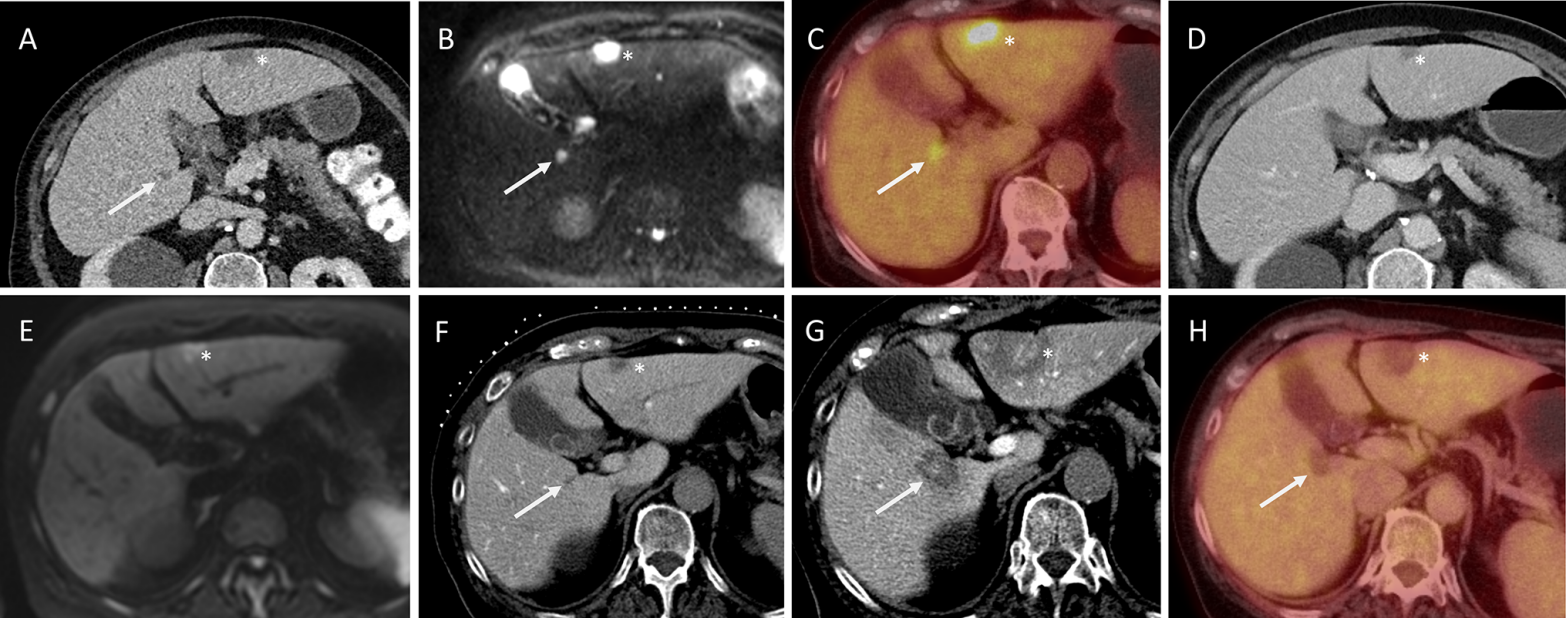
Contrast-enhanced (ce) CT and ceMRI images (A, B) and an 18F-FDG PET-CT image (C) from a 60-year-old men with two colorectal liver metastases in segment III (*) and segment V (white arrow) from a primary rectal carcinoma. After treatment with chemoradiotherapy for the primary rectal carcinoma, the metastasis in segment V disappeared on both ceCT (D) and ceMRI (E). On the intraprocedural CT hepatic arteriography (CTHA), both the metastases in segment III as well as the ‘disappeared’ metastasis in segment V were detected (F) and treated with percutaneous MWA (G) in the setting of the randomized controlled COLLISION trial. Six months after the ablation, there were no signs for local or distant tumour progression on the follow-up 18F-FDG PET-CT (H)
